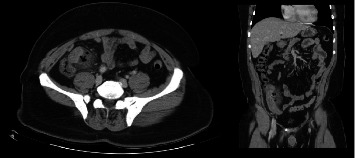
Computed tomography of the abdomen demonstrating mural thickening on the descending and ascending colon with adjacent fatty stranding.
